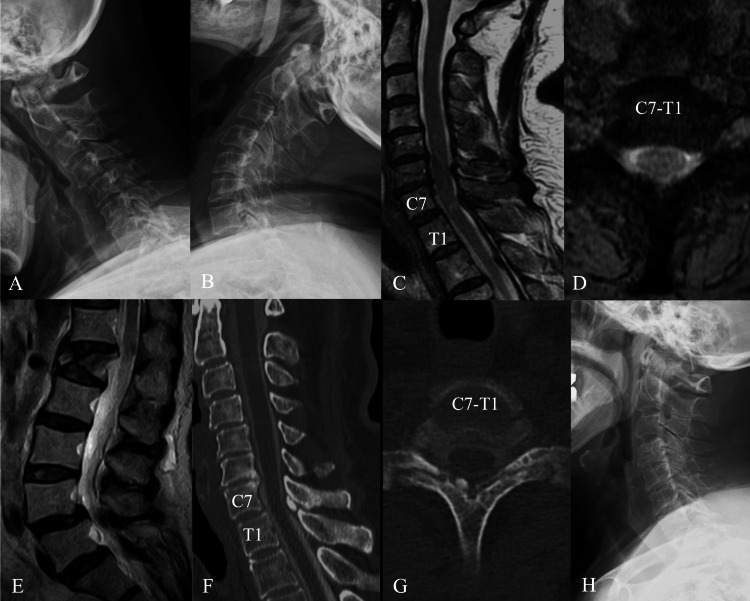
Imaging findings in case 3 (A) Preoperative cervical spine radiograph in flexion lateral view. (B) Preoperative cervical spine radiograph in extension lateral view. There was no instability at the C7-T1 level. (C) T2-weighted sagittal plane magnetic resonance imaging (MRI) showing slightly intramedullary high intensity at the C7-T1 level. (D) T2-weighted axial plane MRI at the C7-T1 level. The spinal cord is compressed posteriorly. (E) T2-weighted sagittal plane MRI of the lumbar spine shows a fracture of the L1 vertebra but no compression of the spinal cord. (F) Paramedian slice of the sagittal computed tomographic myelography (CTM) image showing calcification of the ligamentum flavum on the right side at the C7-T1 level and slight contrast thinning. (G) Axial CTM view showing calcification of the ligamentum flavum. (H) Lateral view of the postoperative radiograph. C7-T1 laminectomy was performed.
